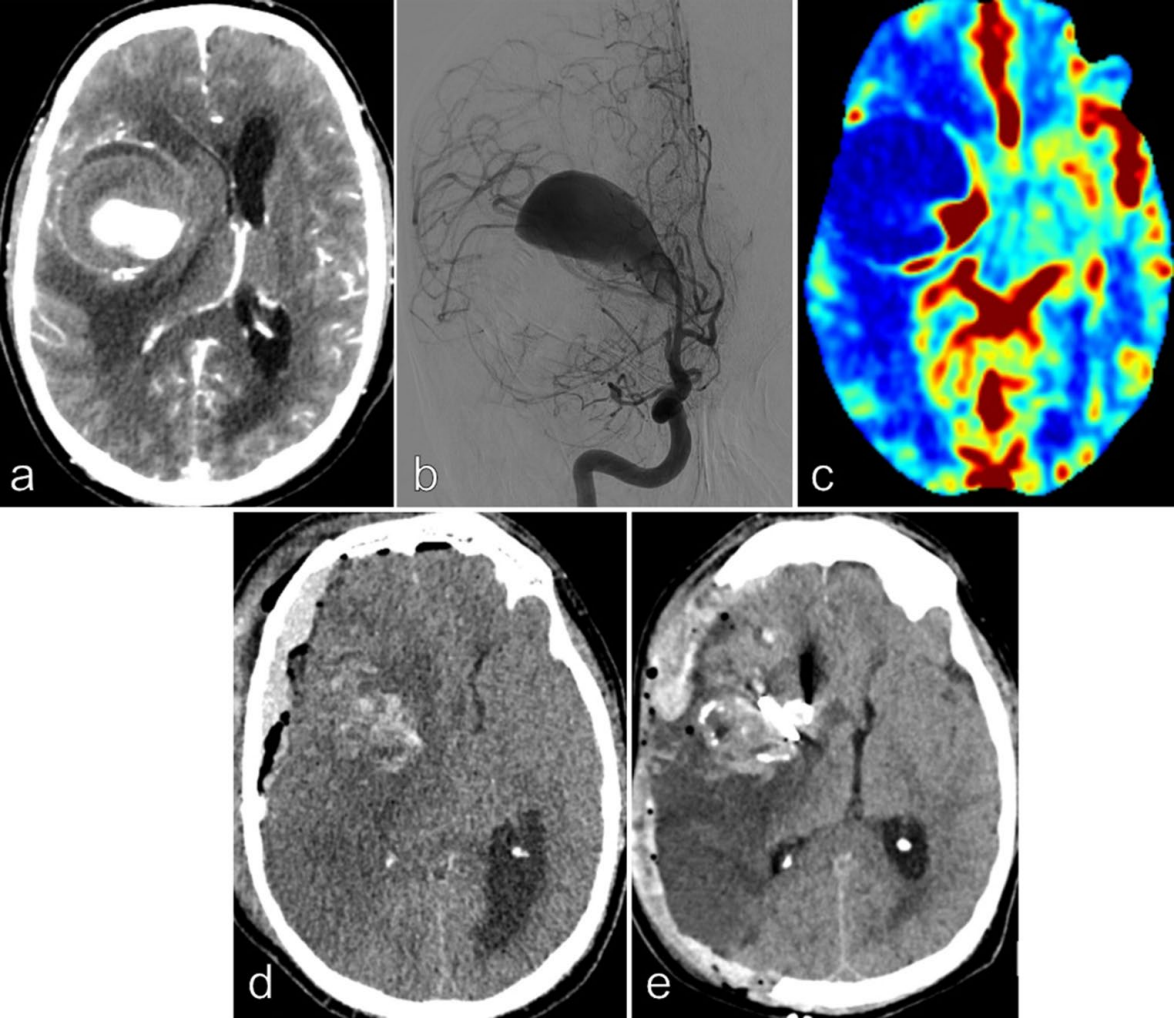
Patient 2, 66 yo male, smoker with a medical history of chronic bronchitis and peripheral arterial occlusive disease of the lower limbs, presented with headache and a 3 weeks history of left moderate hemiparesis. CT scan, MRI/MRA and conventional angiography demonstrated a right giant MCA aneurysm (maximal diameter = 70 mm) injected only by the M1 segment. A significant peri-aneurysmal edema, wall calcifications and intrasaccular thrombus were noted. The midline brain shift was 13 mm (a and b). A severe hypoperfusion in the left distal MCA territory was noted on the perfusion CT scan (c). Double barrel STA-MCA bypass (on the 2 M2 segments) was done, followed by aneurysmal trapping and a partial resection of the sac and thrombus. Twelve hours after treatment, a rapid consciousness disorder with a right mydriasis occurred, related to a right hemispheric diffuse edema and ischemic lesions (d). Decompressive hemicraniectomy was immediately done (e). Fatal evolution with lung infection and scar necrosis were noted. He died 21 days after the treatment
